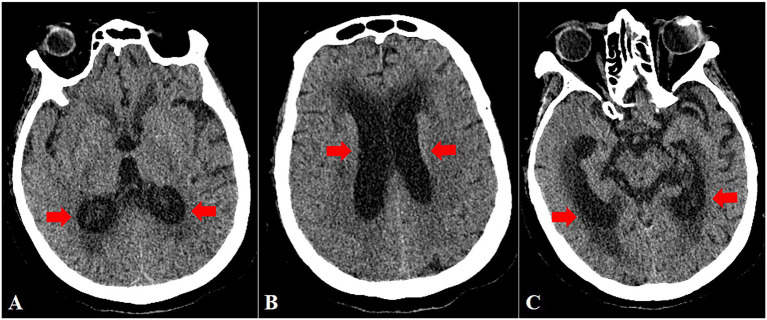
Cranial CT demonstrates bilateral ventricular hydrocephalus. Red arrows indicate dilation of the lateral ventricles.
